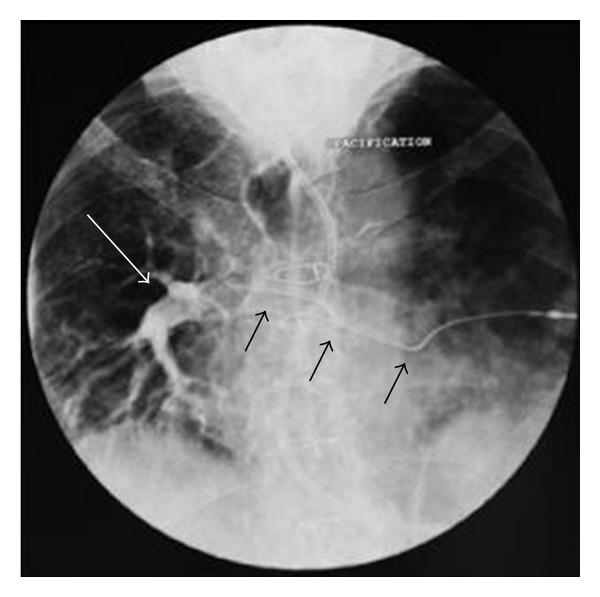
Chest radiography showing an opacification of the right pulmonary artery (white arrow) and the route of the catheter through the 2 pulmonary arteries (black arrows).
